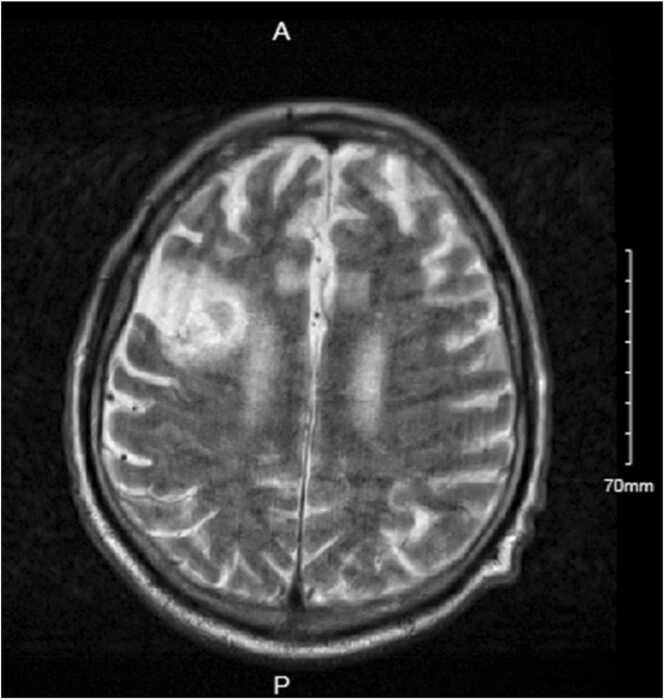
MRI Brain demonstrating a 6 mm ring enhancing lesion concerning for mycotic aneurysm.
